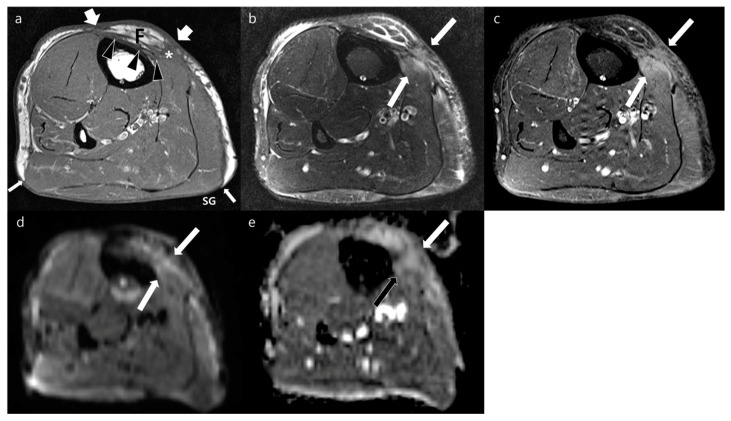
Postoperative MRI obtained from the leg of a 46-year-old woman who underwent surgical resection of myxofibrosarcoma with immediate local flap reconstruction at the anterior tibial region 6 months prior. (a) Axial T1WI shows a flap (F) with peripheral (arrows) and deep (arrowheads) margins on the anteromedial side of the leg. Ill-defined isointense lesion (asterisk) involved deep and peripheral margins of the transplanted tissue. The skin graft (SG) is noted as a thin hypointensity overlying the gastrocnemius muscle. The peripheral margin of the skin graft is indicated by thin arrows. (b) Axial T2WI shows ill-defined nodular hyperintense lesion (arrows). (c) Axial contrast-enhanced FS-T1WI shows moderate enhancement (arrows). (d,e) This lesion (arrows) represents facilitated water diffusion seen as hyperintense signal on axial DWI with a *b*-value of 800 s/mm^2^ (d) and high ADC (e). This lesion decreased in size in follow-up MRI without other treatment; it was then presumed as a postoperative change.
